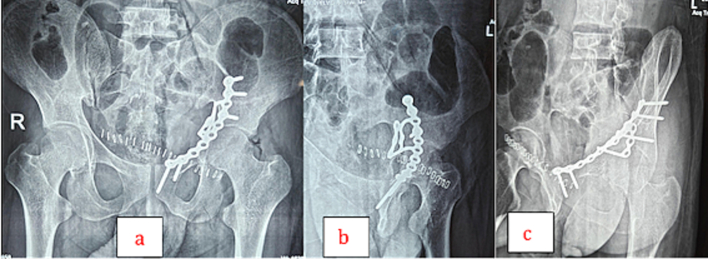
(a) Postoperative X-ray [antero-posterior view]. (b) Postoperative X-ray [iliac oblique view]. (c) Postoperative X-ray [obturator oblique view].
